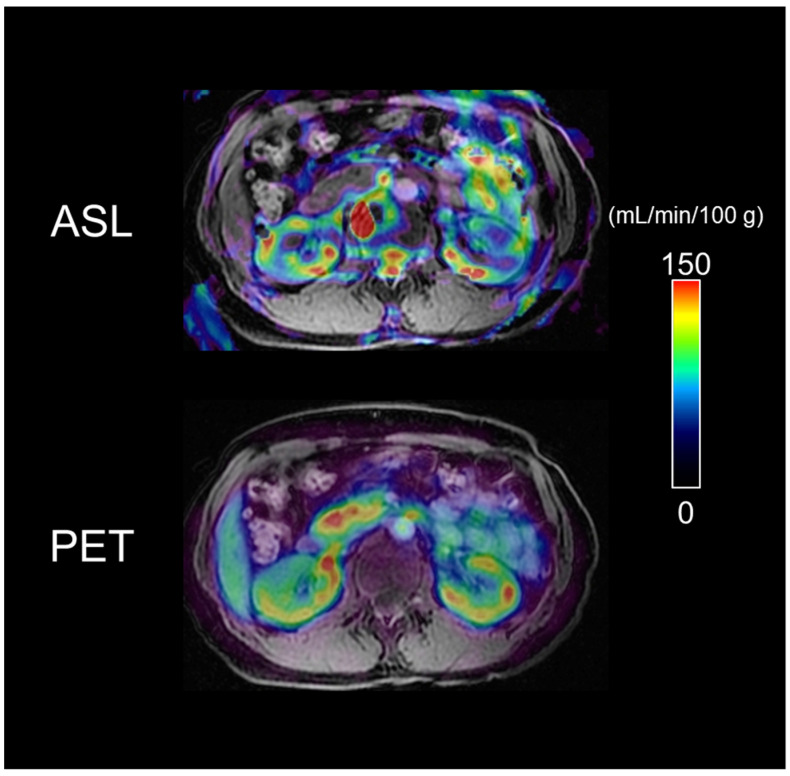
Representative ASL-MRI and ^64^Cu-ATSM-PET images for RBF evaluation in HCs. Both ASL-MRI and ^64^Cu-ATSM-PET allowed visual evaluation of the RBF in the kidney parenchyma. The colour of this scale bar indicates blood flow (mL/min/100 g), with red indicating more blood flow. ASL, arterial spin labeling; MRI, magnetic resonance imaging; PET, positron emission tomography; RBF, renal blood flow; Cu-ATSM, Cu (II)-diacetyl-bis(4-methylthiosemicarbazonate; HC, healthy control.
